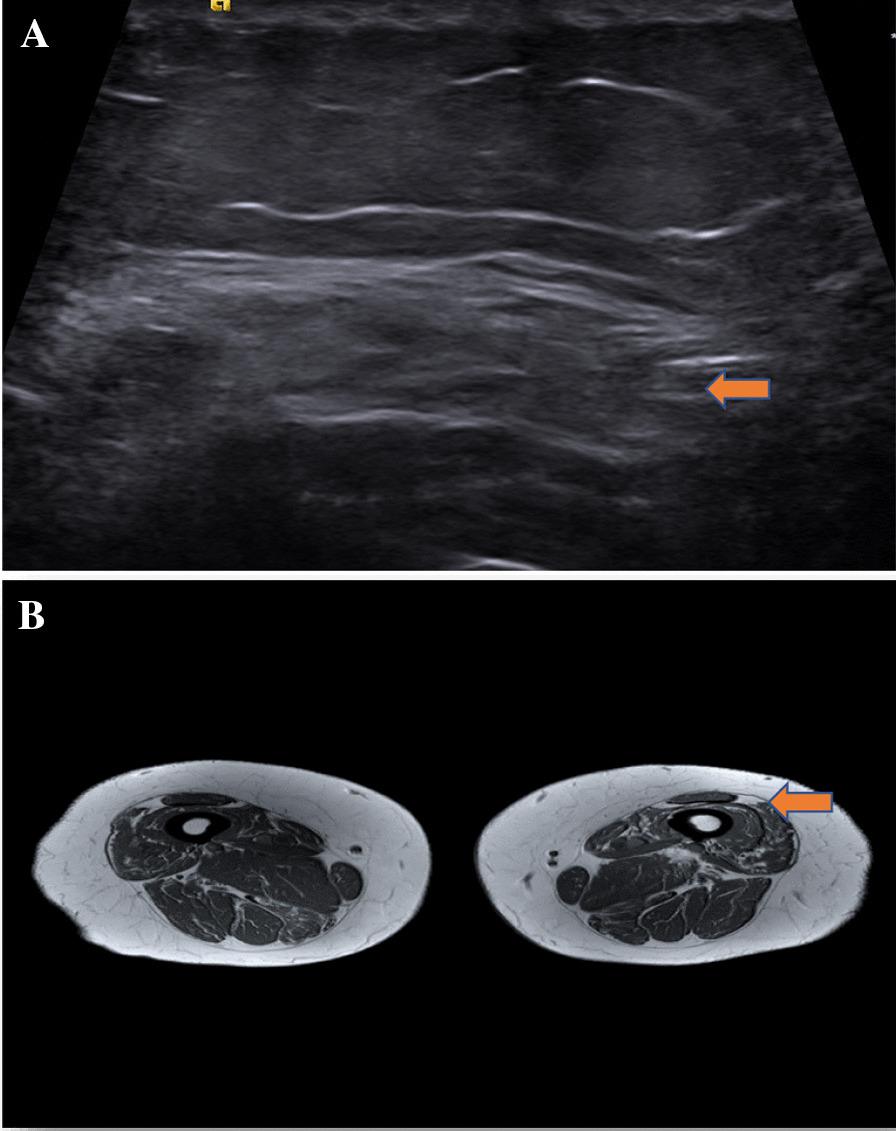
A US showing more heterogenous marked echogenicity in the vastus lateralis in a patient with IBM. B Corresponding MRI showing T1 (not fat-suppressed) uptake in the vastus lateralis in a patient with IBM
